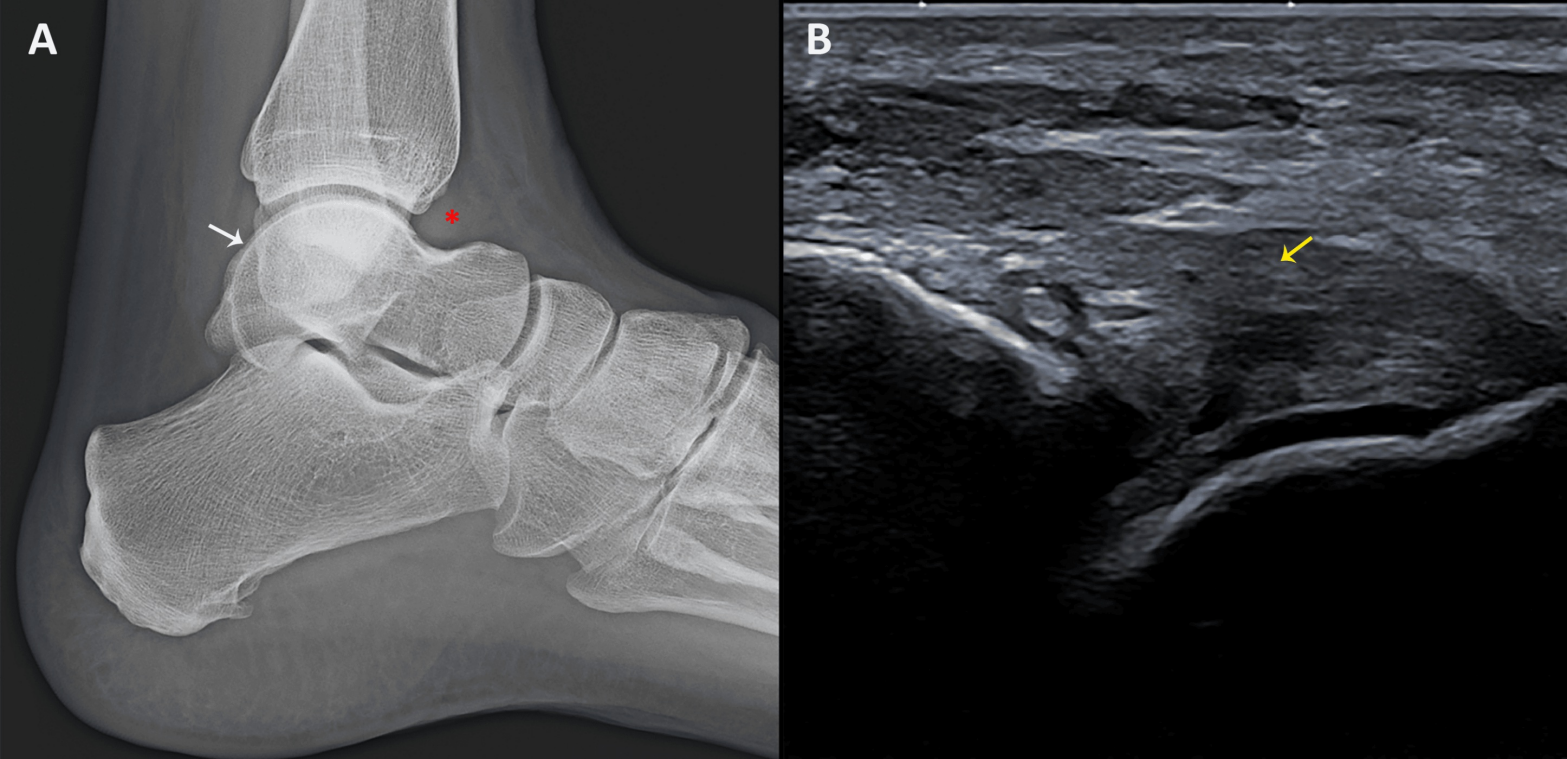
Lateral ankle radiograph and ultrasound image obtained during follow-up evaluation. (A) Lateral radiograph demonstrating satisfactory healing of the subchondral fracture involving the posterosuperolateral aspect of the talar dome (white arrow), as well as persistent joint effusion in the anterior talocrural recess (red asterisk). (B) Longitudinal ultrasound view of the anterior talofibular ligament showing ligamentous thickening consistent with post-sprain sequelae (yellow arrow).
